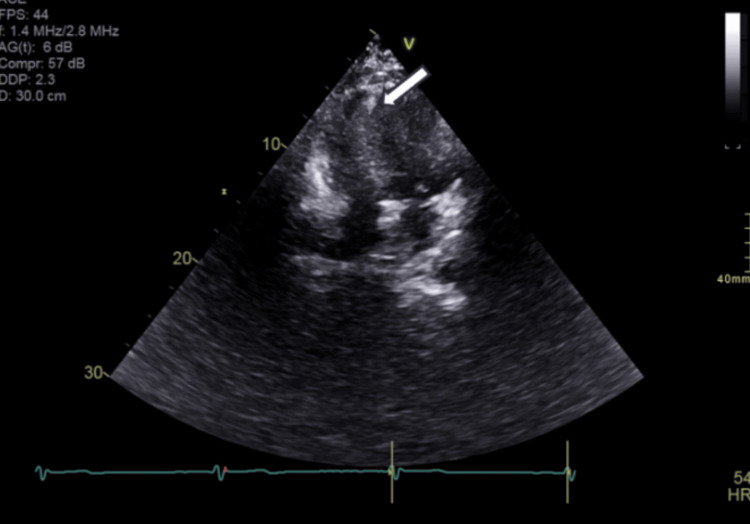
Four-chamber view of the heart on a transthoracic echocardiogram did not identify the cardiac lipoma prospectively. Retrospectively, a hyperechoic area that could represent lipoma is highlighted with an arrow.
